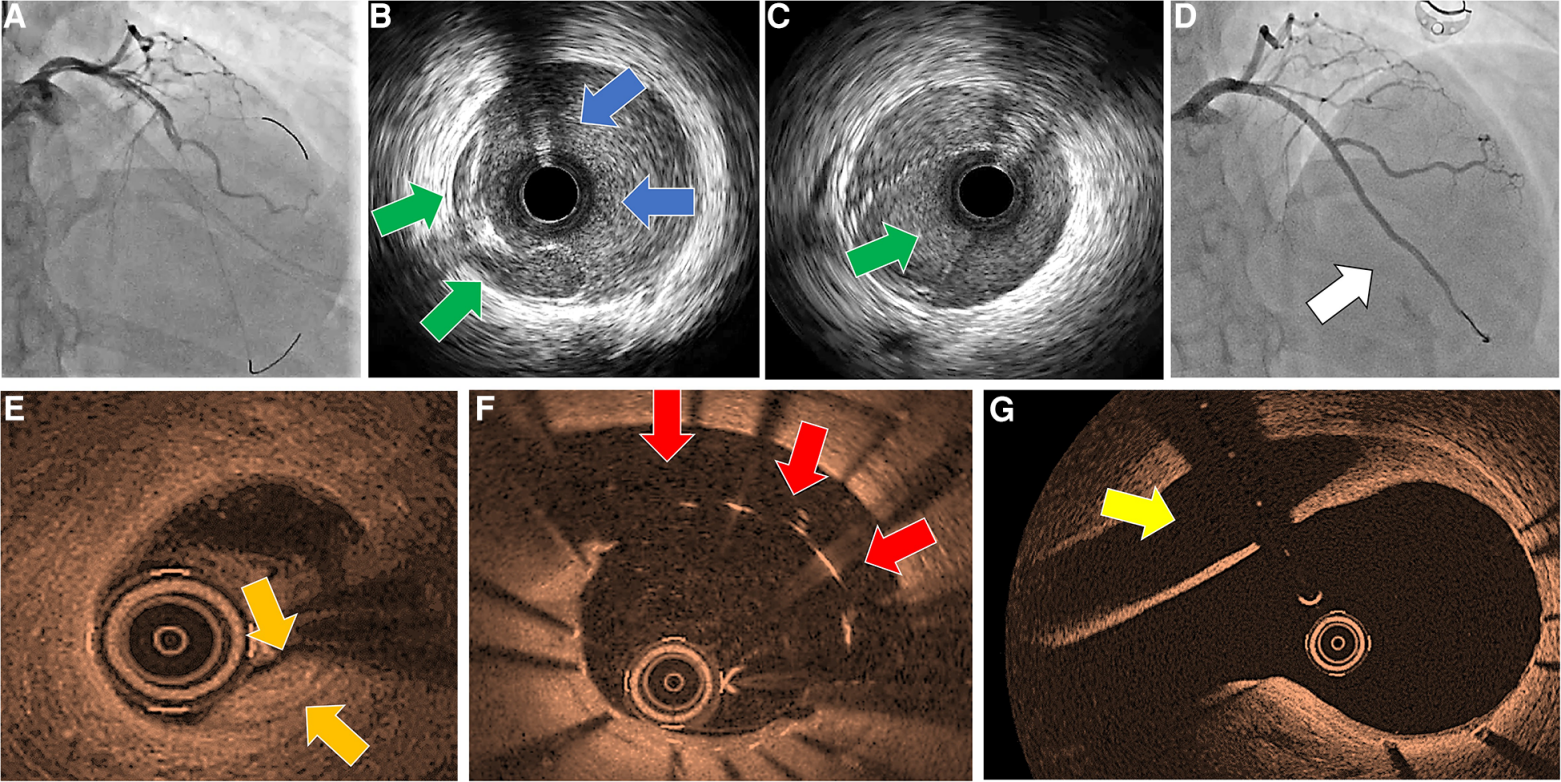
Iatrogenic dissection in an SCAD patient during invasive imaging. (A) First angiographic scene after catheter caused dissection of left-main artery showing distal wide-spreading of dissection; (B) IVUS probe in false lumen (blue arrows), showing compressed true lumen (green arrows); (C) IVUS probe in true lumen (green arrows); (D) result after overlapping stent implantations form left-main to distal left anterior descending; (E) control in-hospital OCT imaging showing compression of true lumen in distal artery (initial SCAD provoking substrate, compressed dissected lumen, orange arrows); (F) stent malapposition with persisting large dissection lumen (red arrows); (G) dissection lumen in left-main artery, caused by catheter engagement (yellow arrow). IVUS, intravascular ultrasound; OCT, optical coherence tomography.
